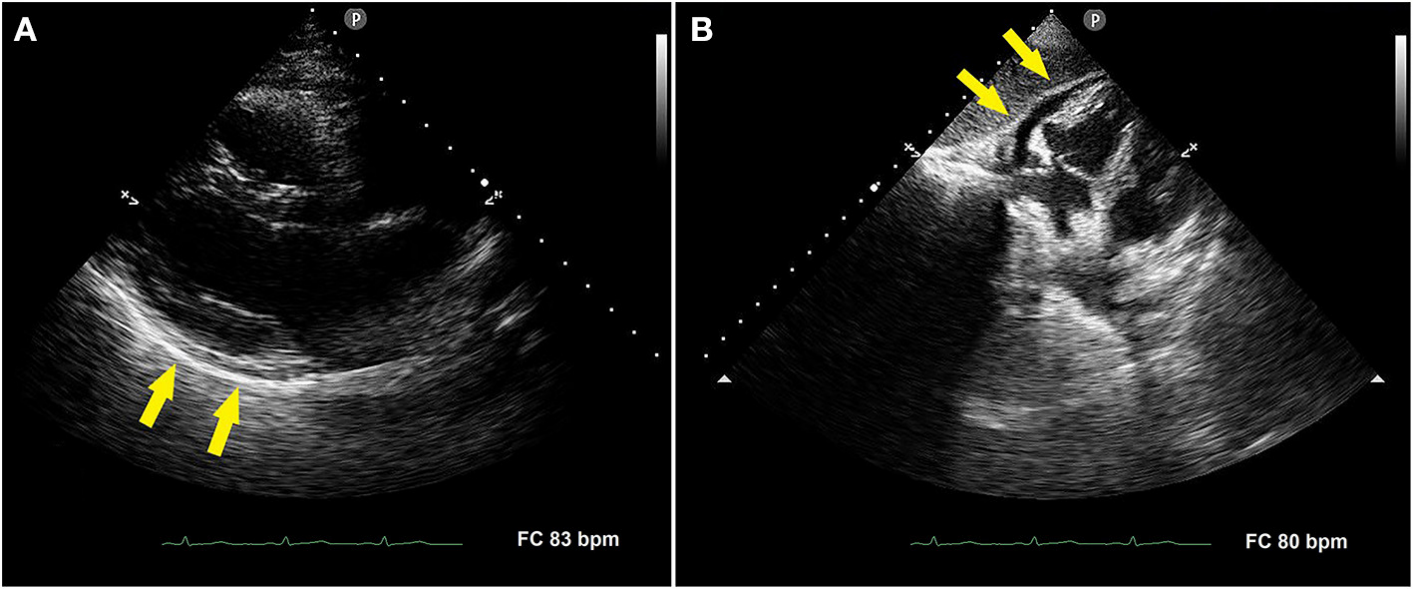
Transthoracic echocardiography performed in the acute phase of disease. Parasternal long-axis view showing mild posterior pericardial detachment [(A), yellow arrows]. Subcostal four-chamber view showing mild anterior pericardial effusion [(B), yellow arrows].
